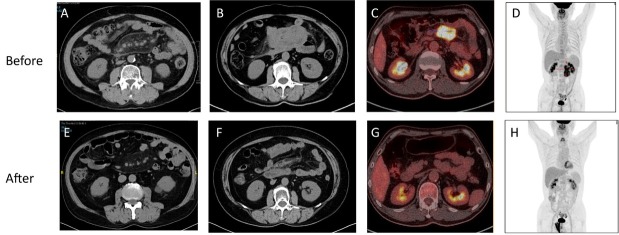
Characteristic imaging findings of IgG4-SM before and after treatment. (A) Abdominal CT axial image of a patient with mild IgG4-SM showing hazy shadows in the mesenteric region. (B) Abdominal CT axial image of a patient with severe IgG4-SM showing irregular soft tissue masses in the mesenteric region. (C-D) PET-CT images of an IgG4-SM patient showing soft tissue masses in the mesenteric region with significantly increased ^18^F-FDG uptake, indicating high metabolic activity. (E-H) Post-treatment images corresponding to (A-D) demonstrate reduced lesion size, resolved hazy shadows, decreased soft tissue masses, and diminished ^18^F-FDG uptake, indicating a favorable treatment response.
